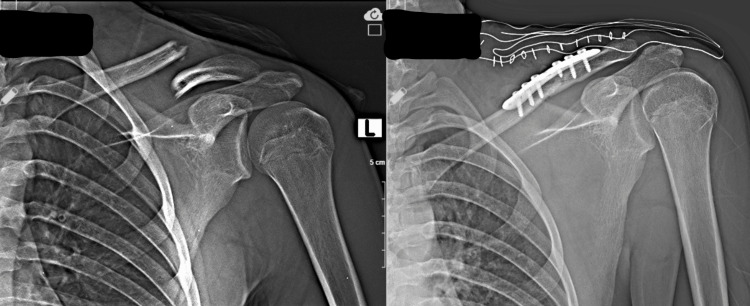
Fifteen-year-old male falls from an e-scooter. The plain radiograph on the left shows a displaced clavicle shaft fracture. Treatment has been made as open reduction and internal fixation. The post-operative radiograph is given on the right.
